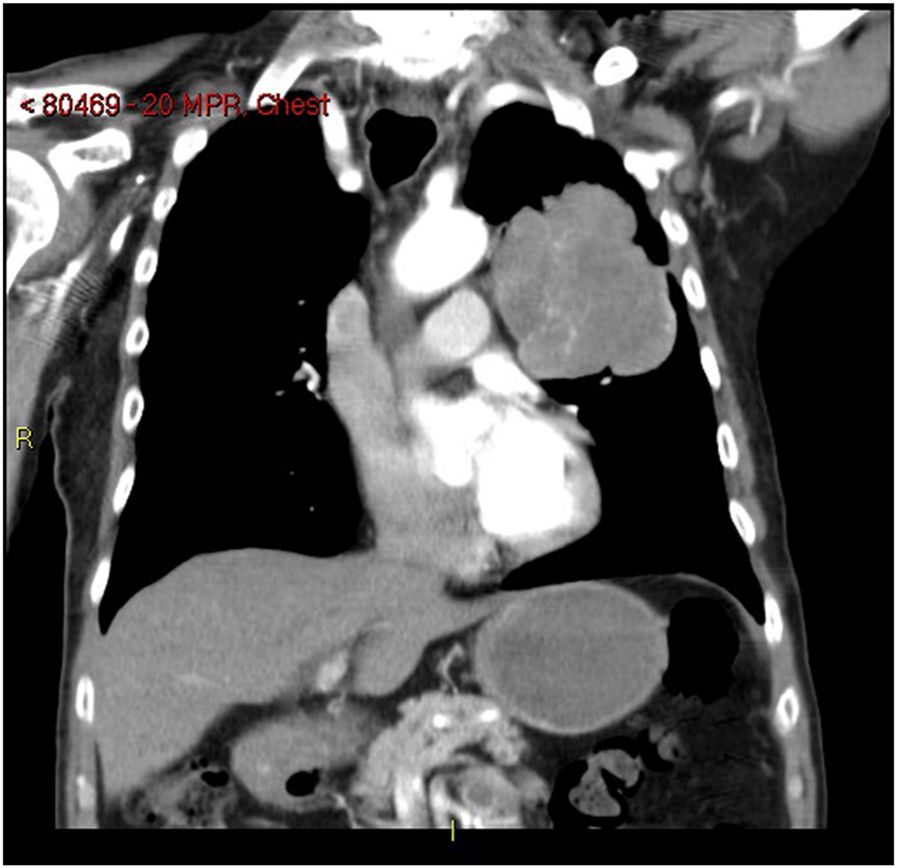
Coronal chest computed tomography slice. This image is a coronal slice of the chest computed tomography taken of the patient. It demonstrates a large squamous cell carcinoma of the lung in the left upper lobe.
